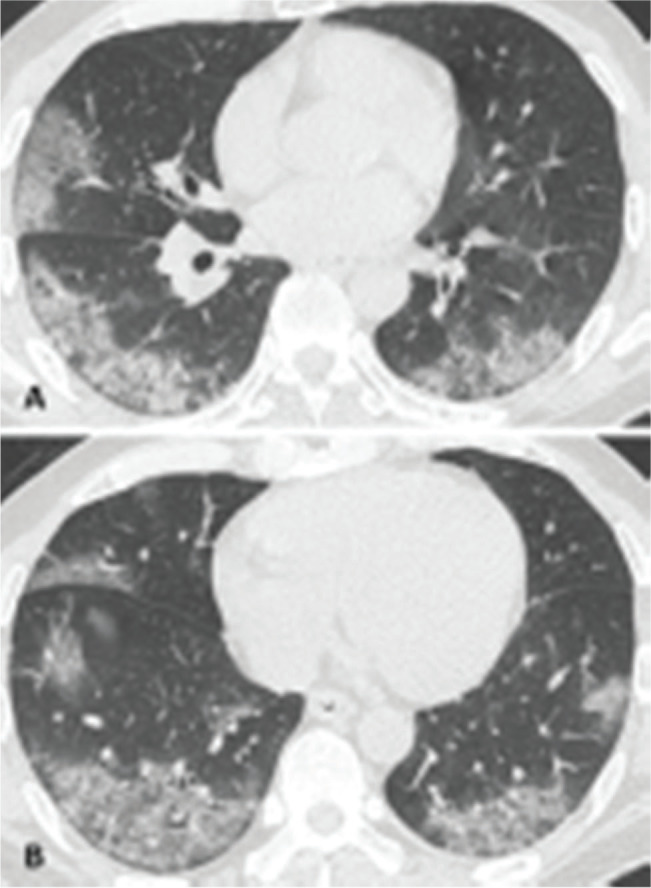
Typical findings of COVID-19. Axial CT images show peripheral, bilateral GGOs with areas of consolidation. Note the associated septal thickening.
